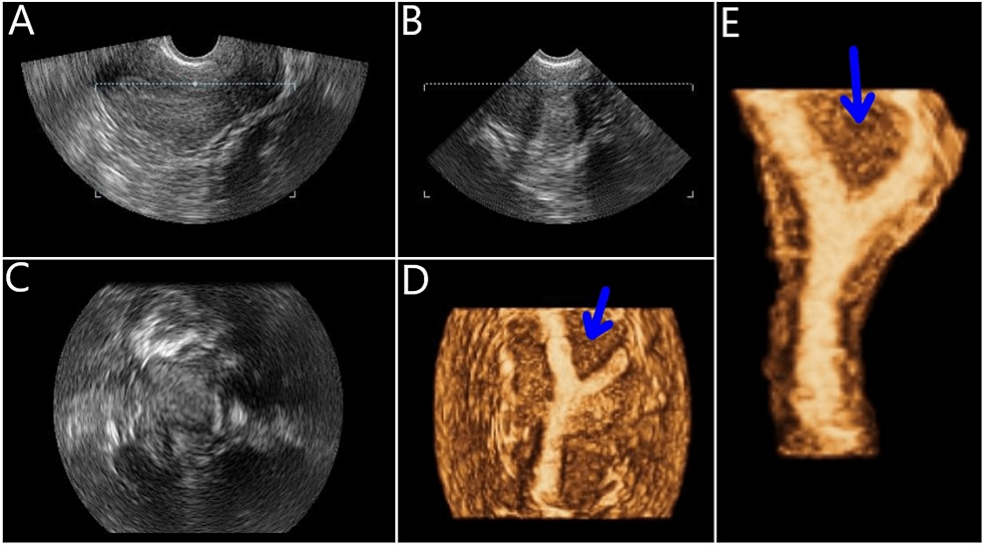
Three-dimensional transvaginal ultrasound scans confirm the clinical suspicion of subseptate uterus. (A) Midsagital plane; (B) Transversal plane; (C) Coronal plane; (D) 3D reconstruction of the uterus; (E) Focused 3D reconstruction of the endometrium
